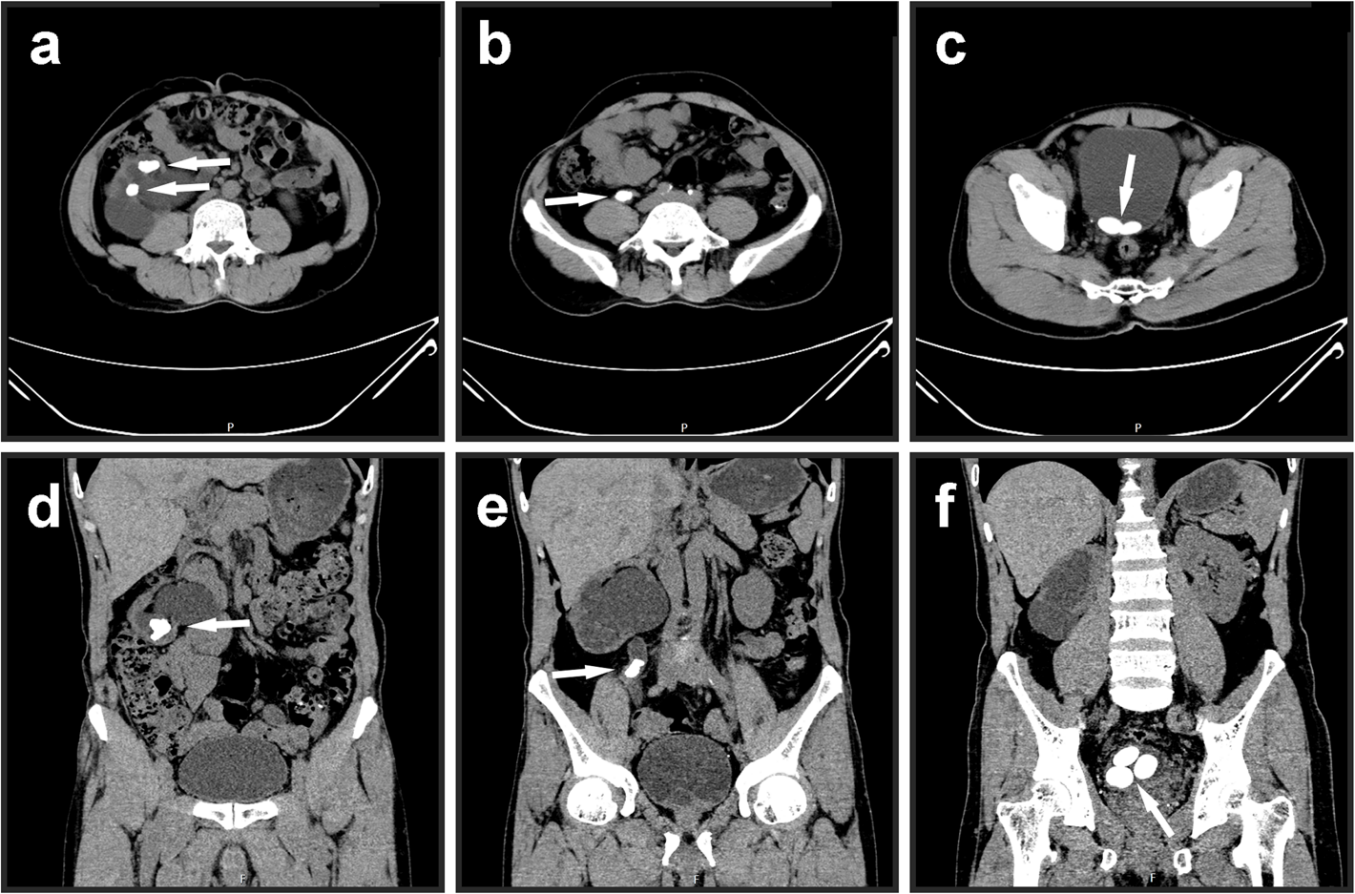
Axial and coronal images of computed tomography showing multiple urinary tract calculi (white arrows). a and d Calculi in the right kidney. b and e Calculi in the right upper ureter. c and f Calculi in the bladder
